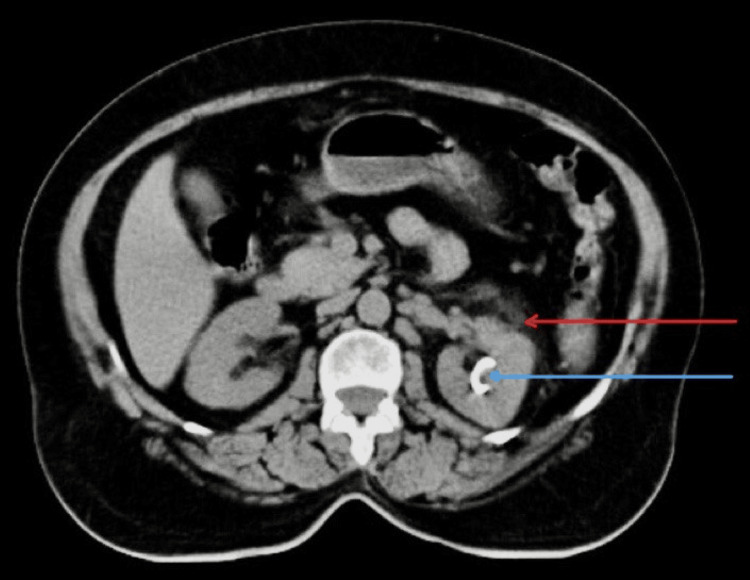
Non-contrast computed tomography of the abdomen (four months post initial scan) showing the left renal parenchyma with urethral stent in-situ (blue arrow) and left-sided perinephric fat stranding (red arrow) with no intrarenal or perirenal air foci noted on the scan.
